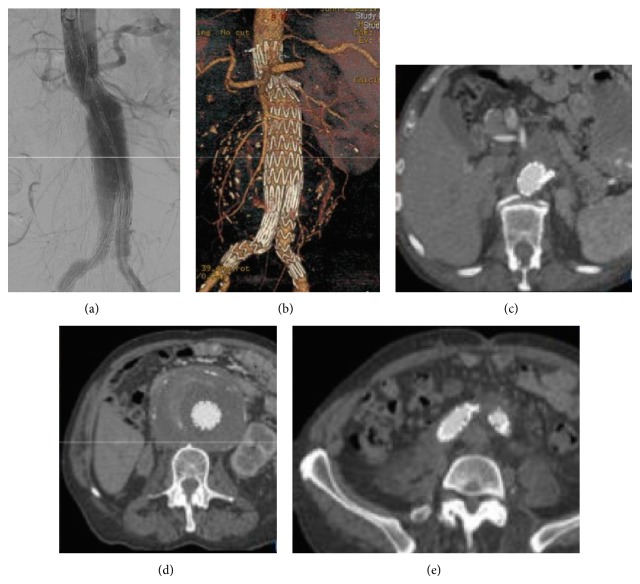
(a) Intraoperative completion angiography showing the good result of the ch-EVAR. (b) Reconstruction of postoperative CTA. Axial views of the postoperative CTA at the level of the origin of the left renal artery (c), mid-aneurysm (d), and bilateral common iliac arteries (e).
